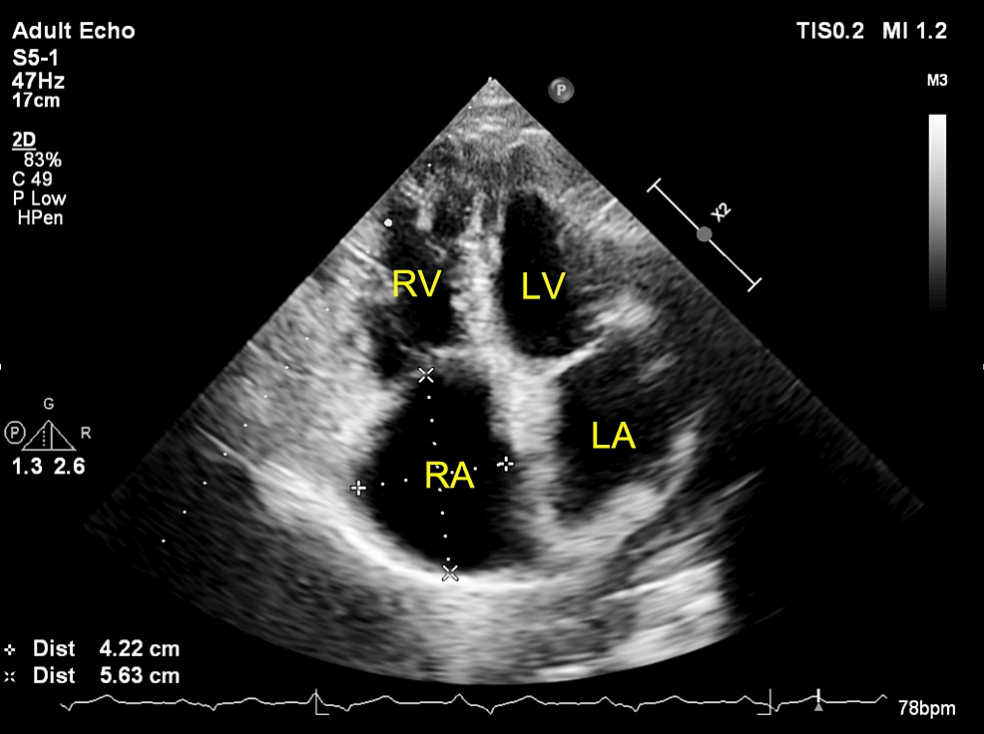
Two-dimensional TTE, apical 4-chamber view, systolic phase, demonstrates a dilated RA. RA: Right Atrium; LA: Left Atrium; RV: Right Ventricle; LV: Left Ventricle.
